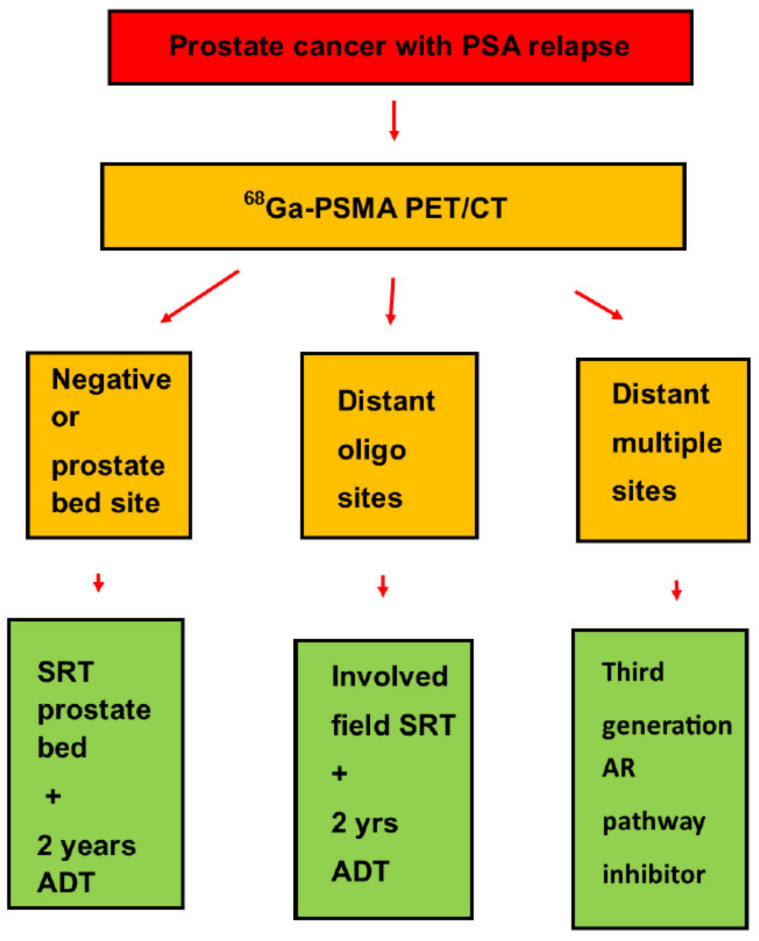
This figure summarizes how ^68^Ga-PSMA PET/CT findings may change treatment decisions for the salvage treatment of patients with PSA relapse
